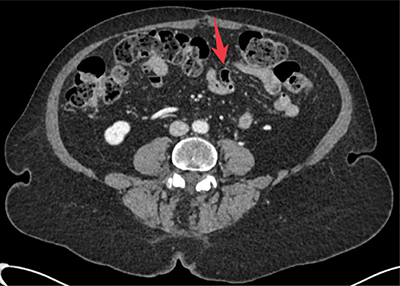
Axial CT image showing jejunal diverticulum from prior examination.
